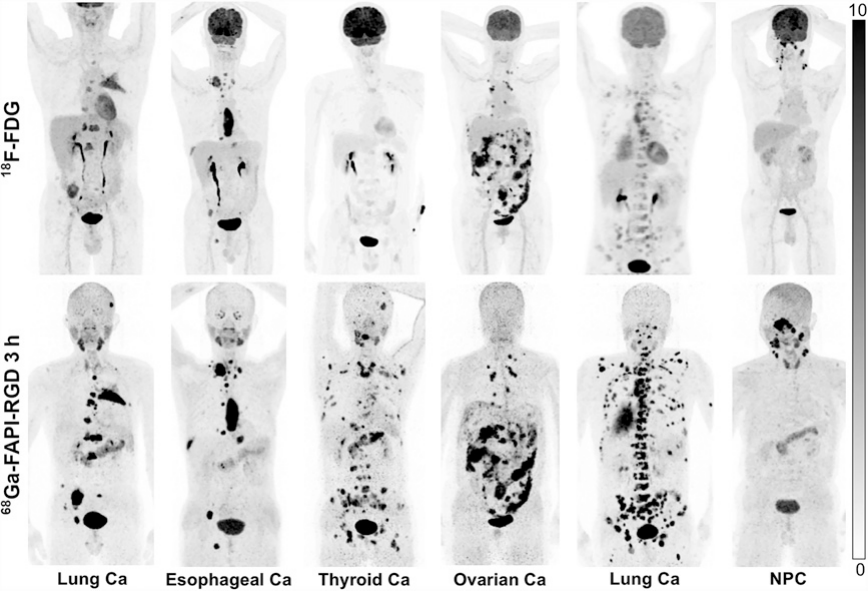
Maximum-intensity-projection images of ^18^F-FDG and ^68^Ga-FAPI-RGD PET/CT in patients with different types of cancer. Ca = cancer; NPC = nasopharyngeal carcinoma.
